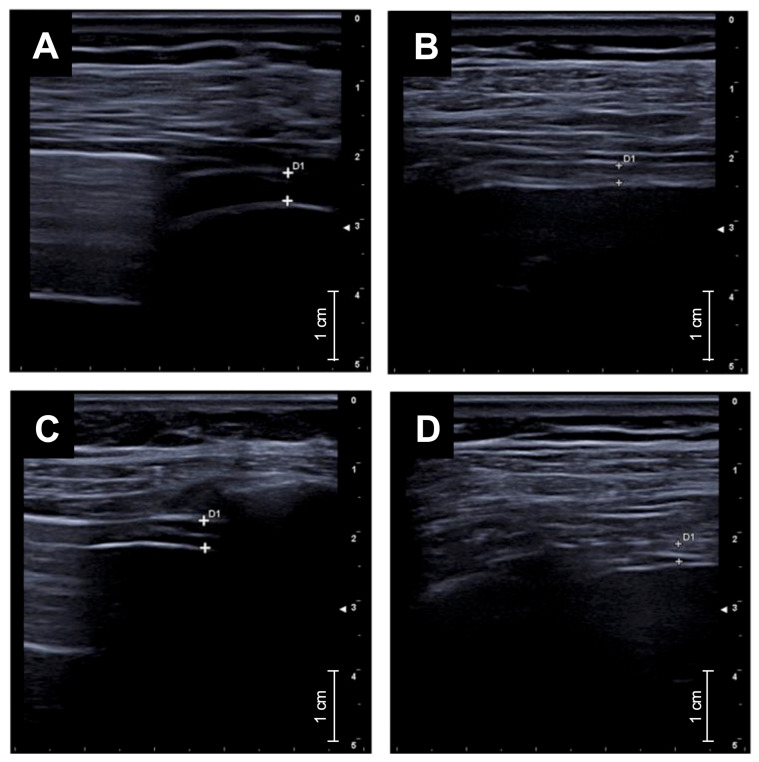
Diaphragmatic ultrasound images showing inspiratory and expiratory thickness at baseline (A,B) and after three months of triple inhaled therapy BDP/FF/G (C,D).
